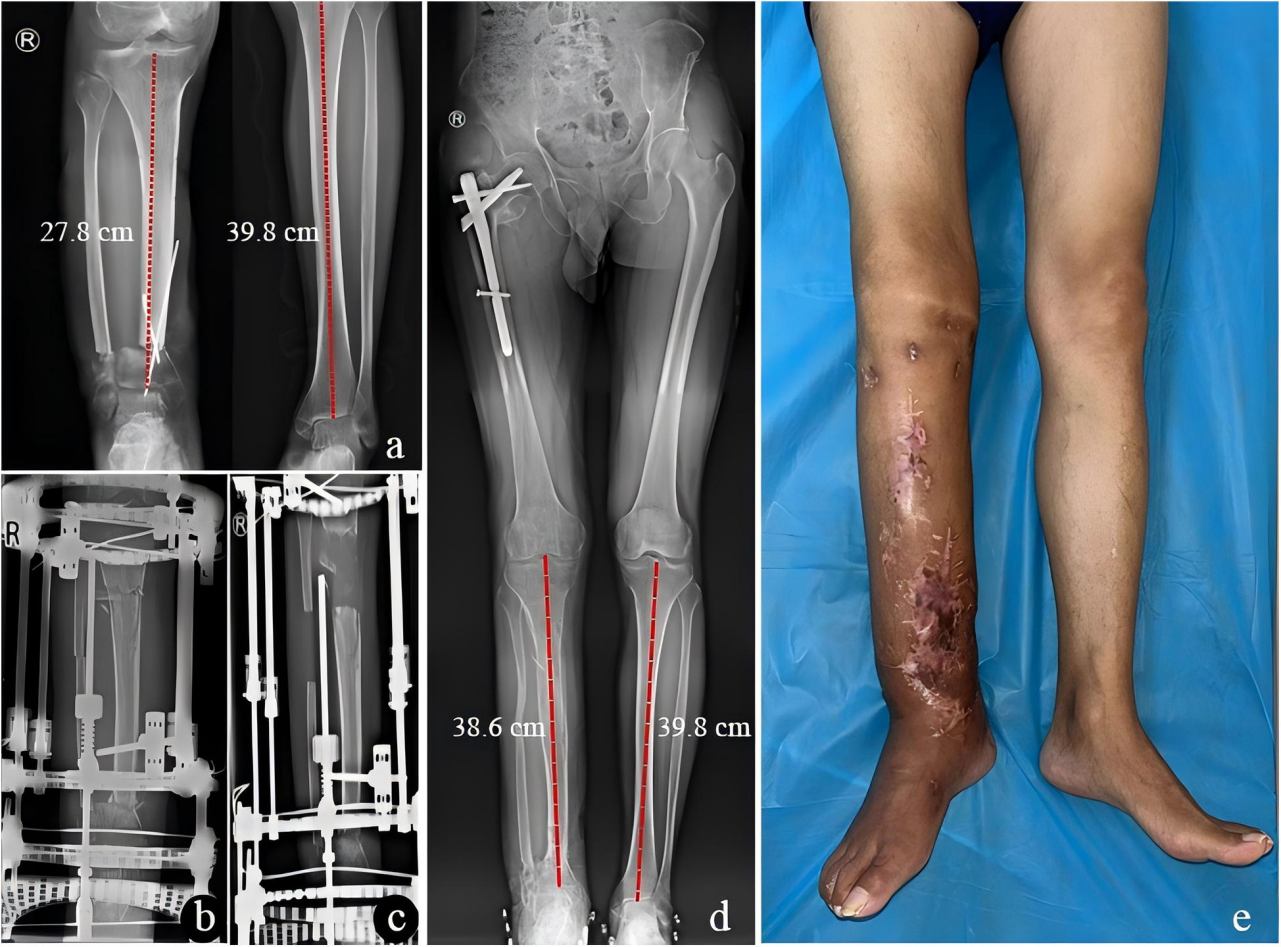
A 37-year-old male with limb shortening deformity after replantation of lower leg amputation treated by limb lengthening. (a) Successful limb salvage and replantation of the affected limb after shortening approximately 12 cm. (b) x-ray of the right tibia before limb lengthening. (c) Three months after limb lengthening. (d,e) The implantation of the Proximal Femur Bionic Nail (PFBN) in the proximal femur for initial intertrochanteric fracture may not affect the overall study findings, as it had no impact on the primary endpoint of limb length discrepancy correction or the functional outcomes based on tibial measurements. The consolidation phase was completed with satisfactory bone and functional outcomes after 19 postoperative months.
